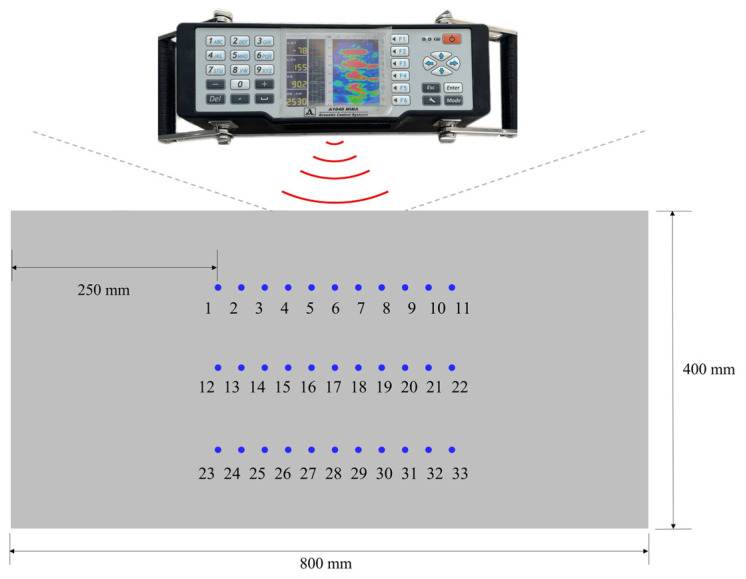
The location of defects for ultrasonic signal collection.
